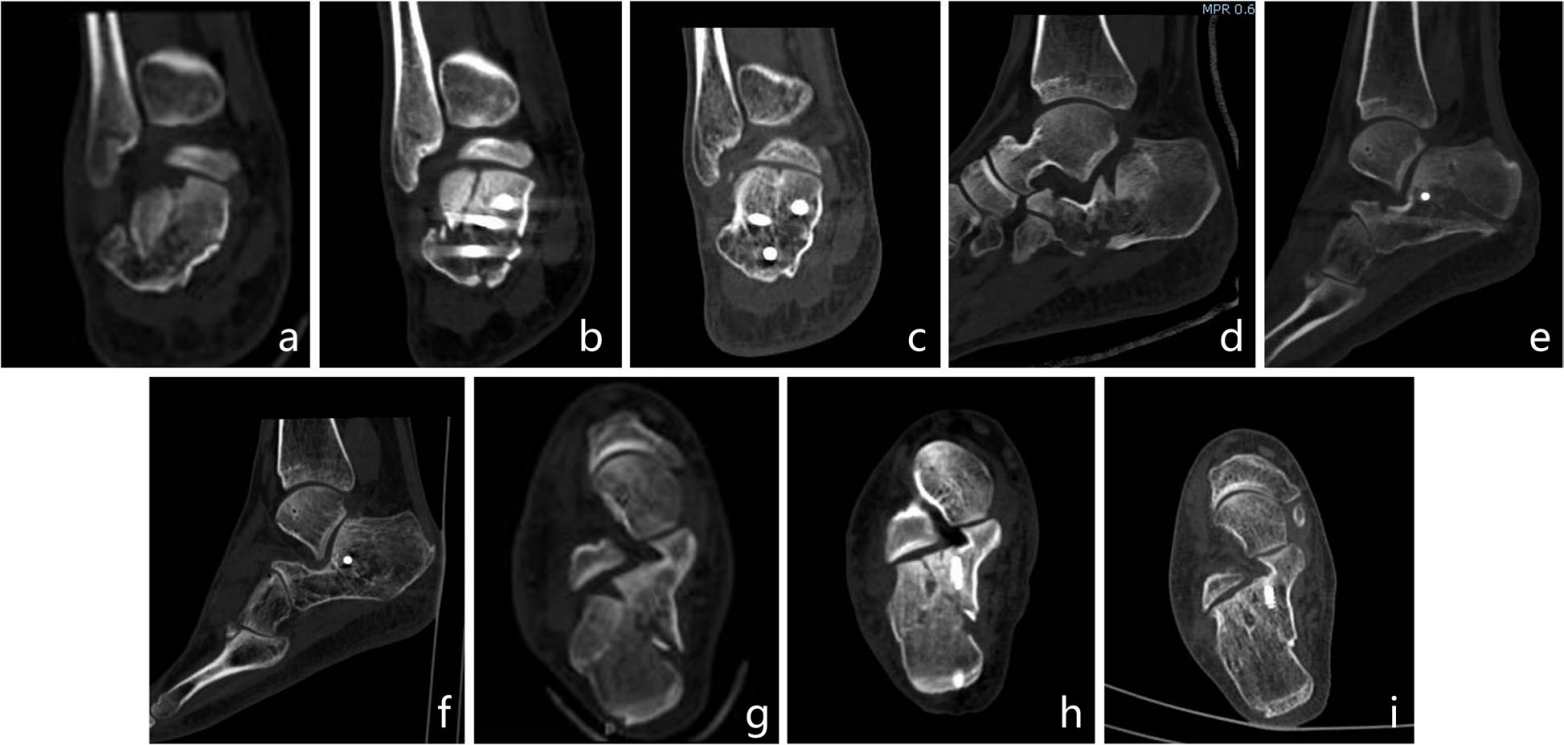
A 37-year-old male patient, Essex-Lopresti classification (joint compression type), Sanders classification (II B). Preoperative CT: coronal (a), sagittal (b) and axial (c) views showed significantly decreased calcaneal height and BÖhler angle. Immediate postoperative CT:coronal (d), sagittal (e) and axial (f) views showed calcaneal anatomical reduction,sagittal (e) view showed a cavity has been present in the neutral triangle after reduction.17 months postoperative CT:coronal (g), sagittal (h) and axial (i) views showed fracture has healed without reduction lose, sagittal (h) view showed the cavity has been filled with massive cancellous bone
